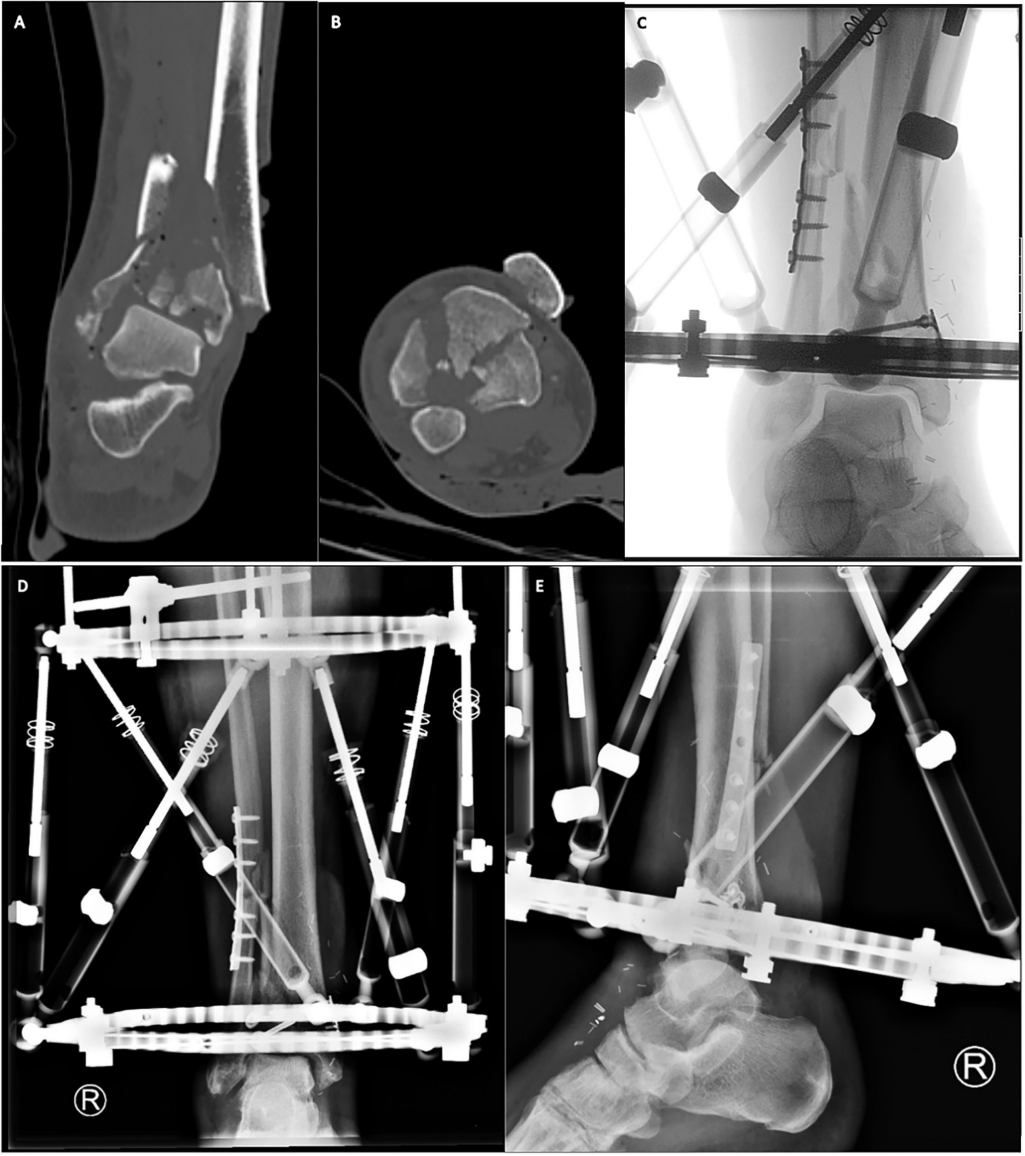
Primary Hybrid external fixation in combination with plate and lag screw osteosynthesis in a °II open tibial pilon fracture. We present a 55-year-old male, with a °II open tibial pilon fracture AO type 43-C3, who presented via the trauma room. After emergency preoperative CT scans [(A) coronal plane, (B) axial plane] temporary fixation and soft tissue conditioning was performed. The extensive soft tissue defect on the anteromedial side was covered with an arteria radialis microvascular free flap. Afterwards the comminuted fibula was restored in length and rotation and the articular surface of the tibial pilon was reconstructed using minimally invasive plating and lag screws [(C) Intraoperative fluoroscopy]. Finally, we stabilized the tibial pilon fracture using a hybrid circular frame with tensioned K-wires in the distal fragments and Schanz-screws in the proximal tibia [(D) Post-operative a.p. X-ray, (E) Post-operative lat. X-Ray].
